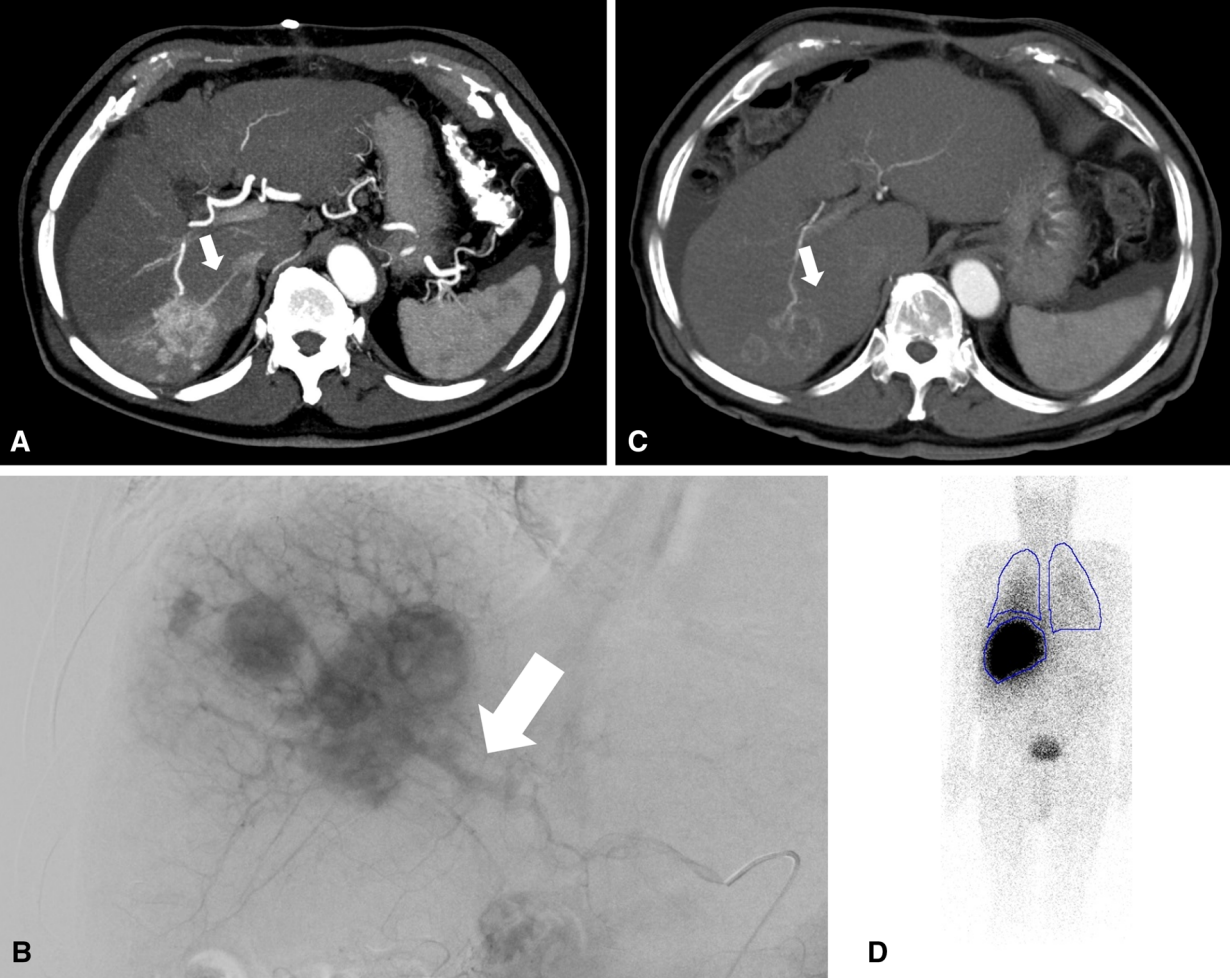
An 82-year-old patient with multifocal HCC in the right liver lobe due to hemochromatosis. A An arteriovenous fistula (white arrow) can be delineated based on the CT images. B DSA confirms the large arteriovenous fistula (white arrow) with high-flow conditions. C After treatment with sorafenib, a significant fistula cannot be visualized anymore. Although the portal vein is slightly contrasted, that might be caused by contrast through sinusoidal collaterals. In DSA no correlate for the previous fistula was found. Of note, even after short-term sorafenib therapy, HCC exhibits devascularization and central necrosis can be seen (white arrow). D MAA administration was carried out, and SPECT reveals a low HPS fraction with 9.9% (lung and liver are delineated in thin blue lines)
